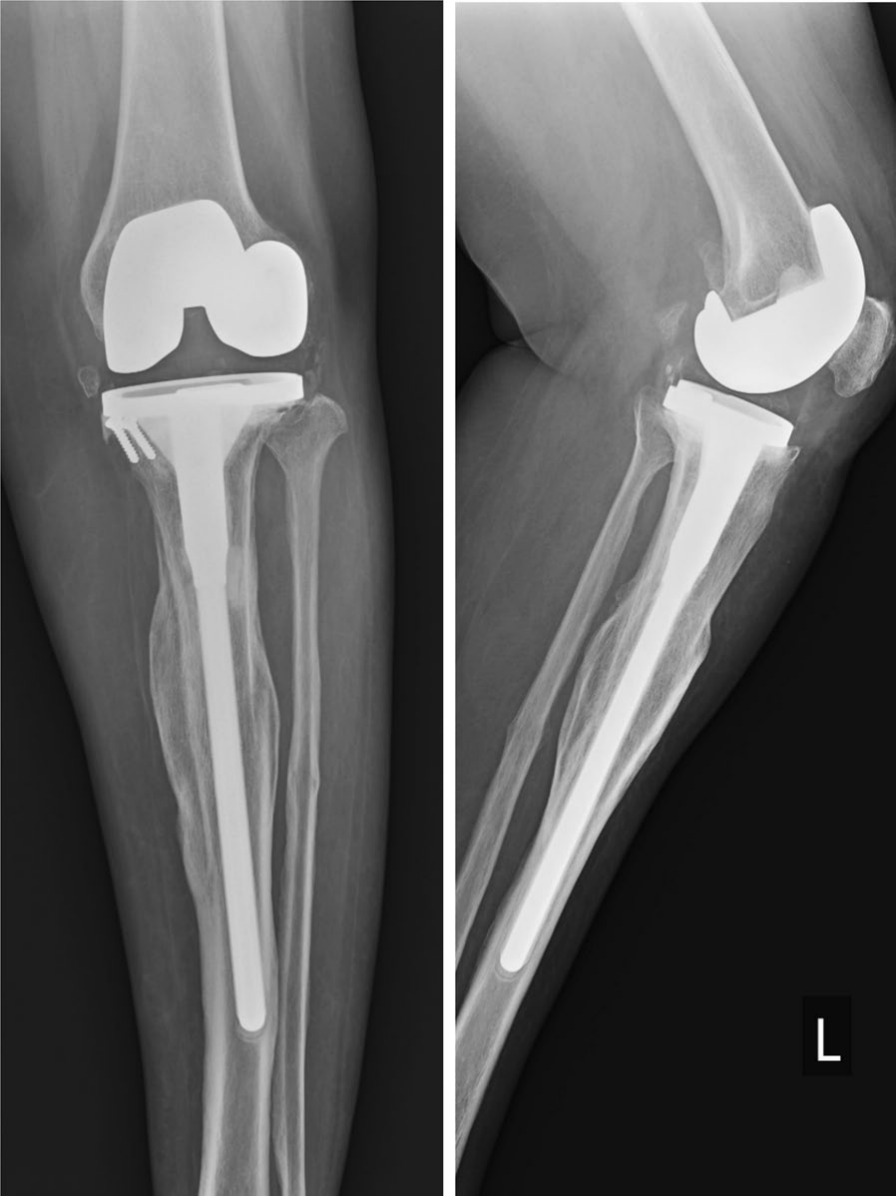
Radiograph of the case 1 patient at 5-year follow-up showing solid union of extraarticular fracture. The patient did not have any complaints, though a radiolucent line is seen just below the lateral tibial plate
